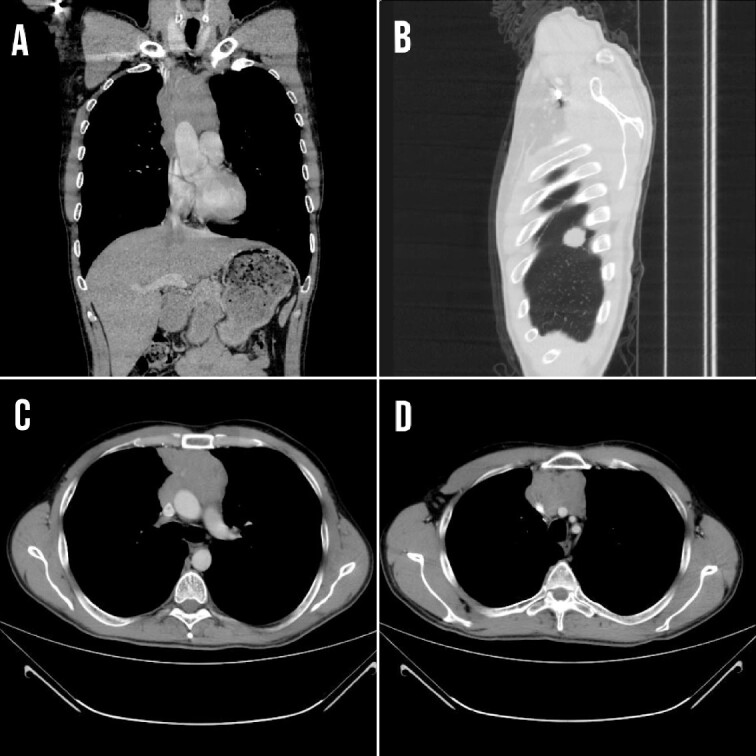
(A) CT coronal section showing the mediastinal lesion. (B) Right diaphragm metastasis at the posterior costal margin at the level of 4 and 5 thoracic ribs (C and D) Transverse section demonstrating the mass that compressed and invaded the major neck veins and the SVC.
